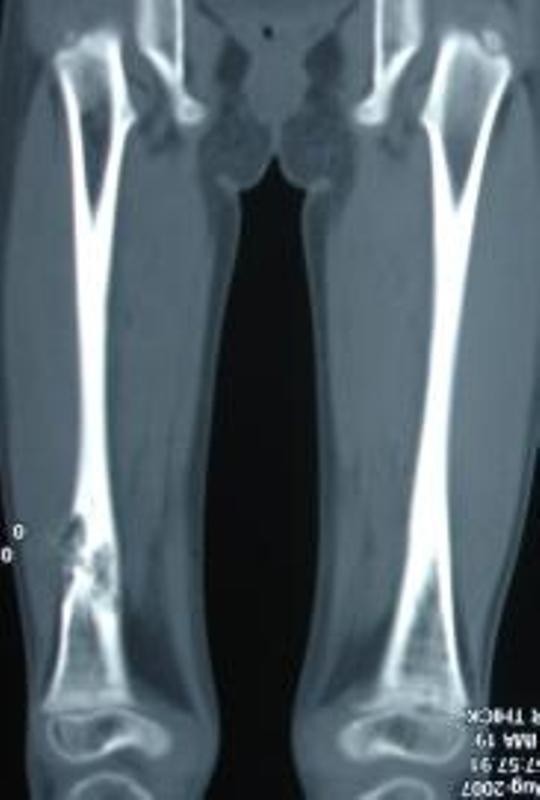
A coronal computed tomography shows osteolytic lesion in the lower third of right femur.
